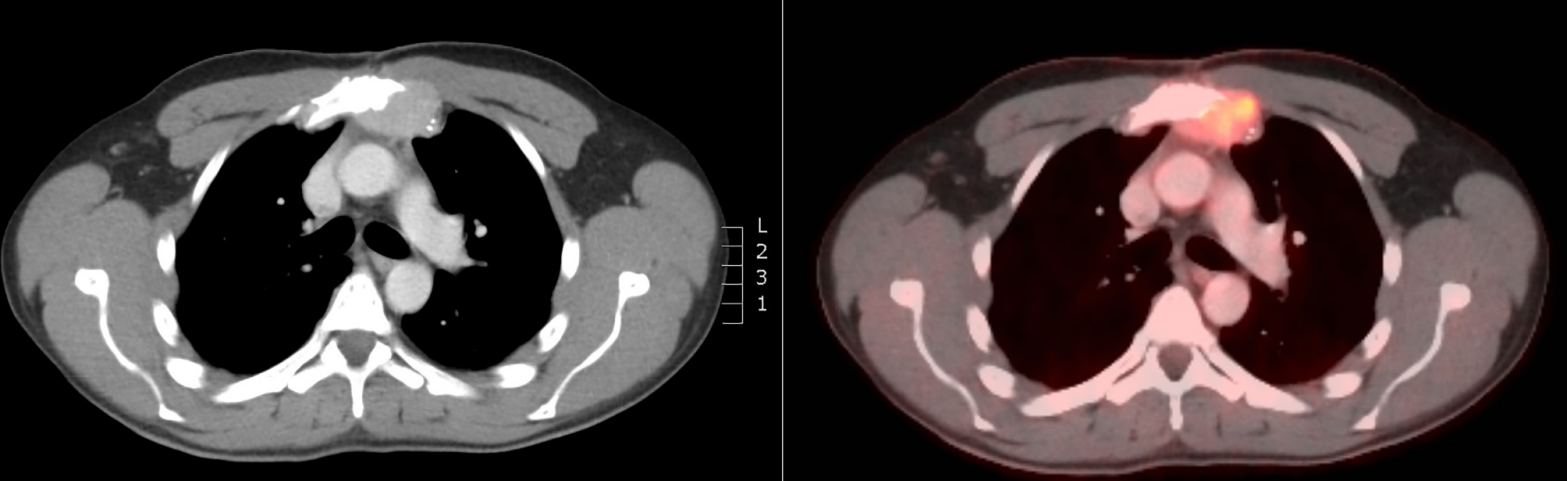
FDG PET/CT at diagnosis of inoperable retrosternal relapse with bone invasion.
